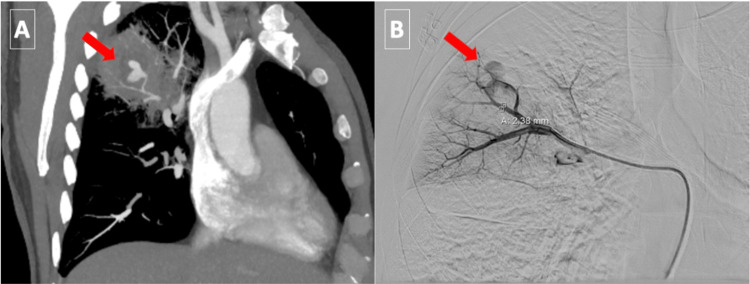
CTA chest A: CTA chest right pulmonary artery oblique maximum intensity projection (MIP) view demonstrating hyperdense focus of contrast extravasation and Rasmussen aneurysm (red arrow). B: Angiography of the segmental pulmonary artery to the right upper lobe demonstrating a Rasmussen aneurysm (red arrow). CTA: computed tomography angiography.
